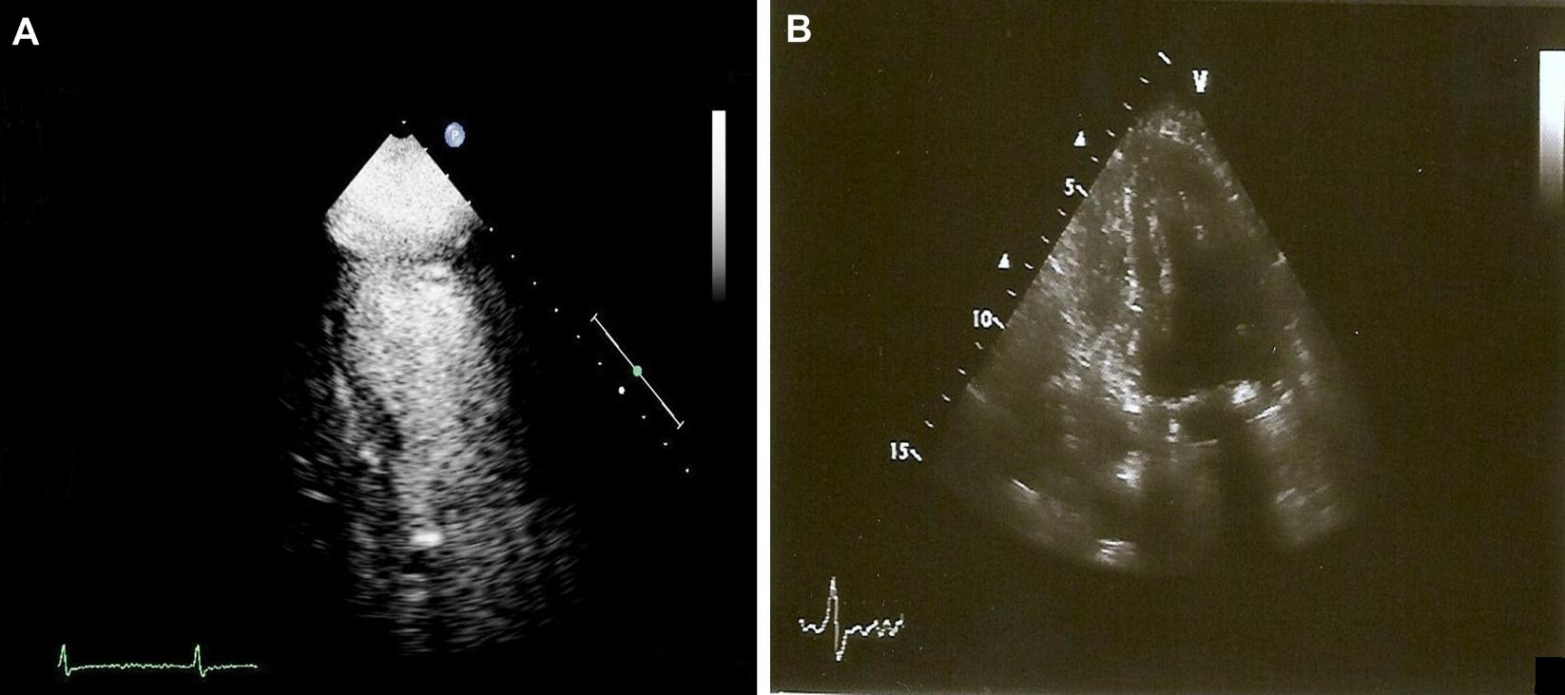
Echocardiogram on initial presentation and follow-up. (A) Echocardiogram with contrast enhancement revealing apical ballooning during the patient's first presentation to our hospital. (B) Echocardiogram revealing normally contracting apex with complete recovery of ejection fraction one month after the patient's initial presentation.
